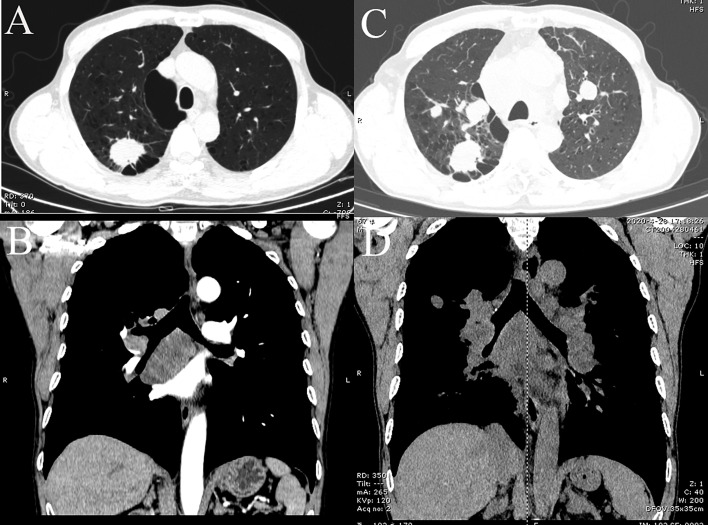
CT images of lung tumor and mediastinal lymph nodes of patient. (A, B) Baseline inspection on April 18, 2019, showed right upper lobe tumor and subcarinal lymph node metastasis, respectively. (C, D) Images on April 6, 2020, suggested multiple pulmonary metastases and multiple mediastinal lymph node metastases, respectively.
